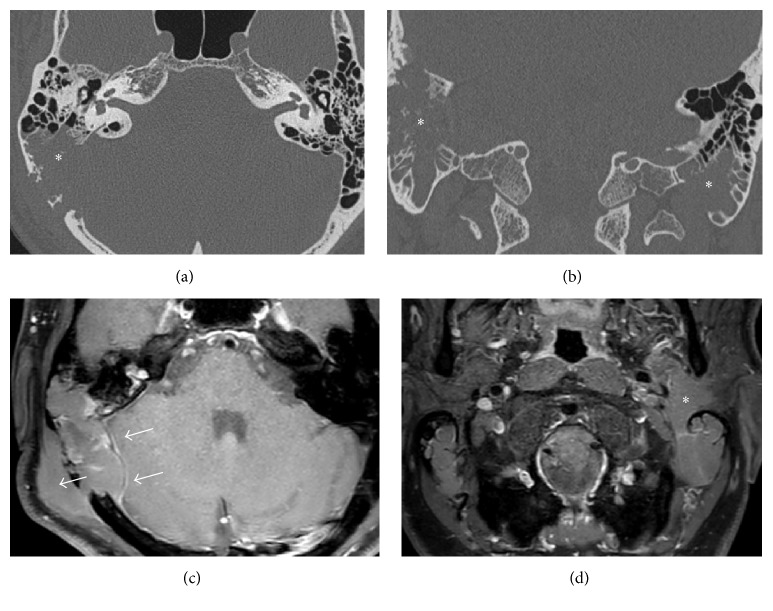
Axial (a) and coronal (b) HRCT images reveal extensive permeative destructive lesions involving both temporal bones (white asterisks). Axial postcontrast fat suppressed T1W axial (c) and coronal (d) images reveal postcontrast enhancement of large associated subgaleal and extracranial intradural soft tissue mass (arrows) on the right side and a moderately large lobulated soft tissue mass along undersurface of left temporal bone (asterisk) causing compression of the extracranial segment of left facial nerve.
